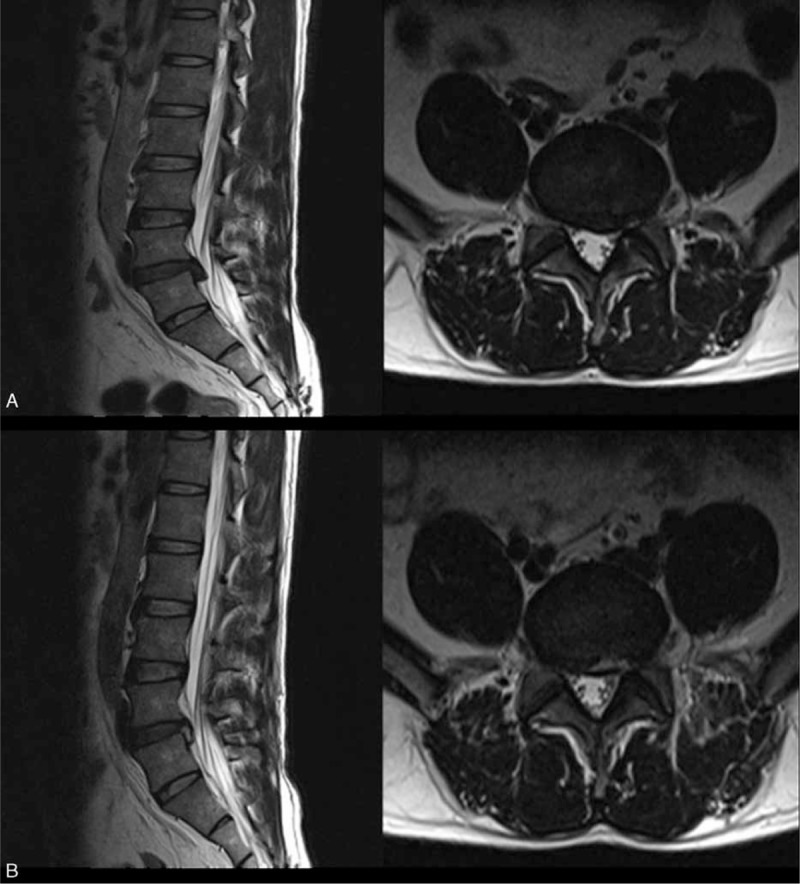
Comparison of magnetic resonance images before (A) and after (B) operation. (A) Preoperative sagittal and axial images showing a highly down-migrated herniation. (B) Postoperative sagittal and axial images of the same patient showing complete removal of the migrated fragments at the 1 month after surgery.
